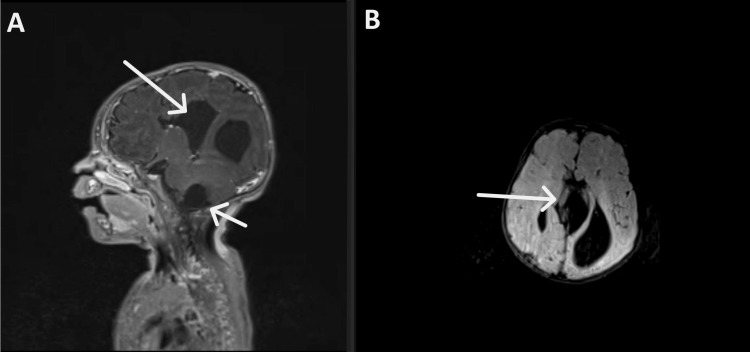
(A) Sagittal T1-weighted post-contrast MRI showing an interhemispheric cyst and a cyst in the posterior part of the medulla oblongata. (B) Axial T2-FLAIR (fluid-attenuated inversion recovery) MRI demonstrating the interhemispheric cyst and agenesis of the corpus callosum.
